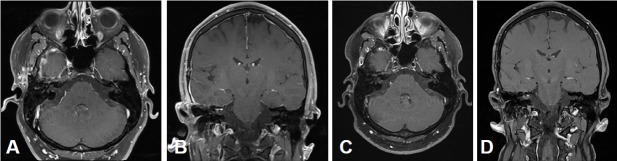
MRI, T1 FS + CM; A, C axial; B, D coronal; A, B 3 months after surgery; C, D 5 years after surgery. No hint for residual or recurrent tumor in the internal auditory meatus on the right side. Postoperative changes in the right temporal region.
